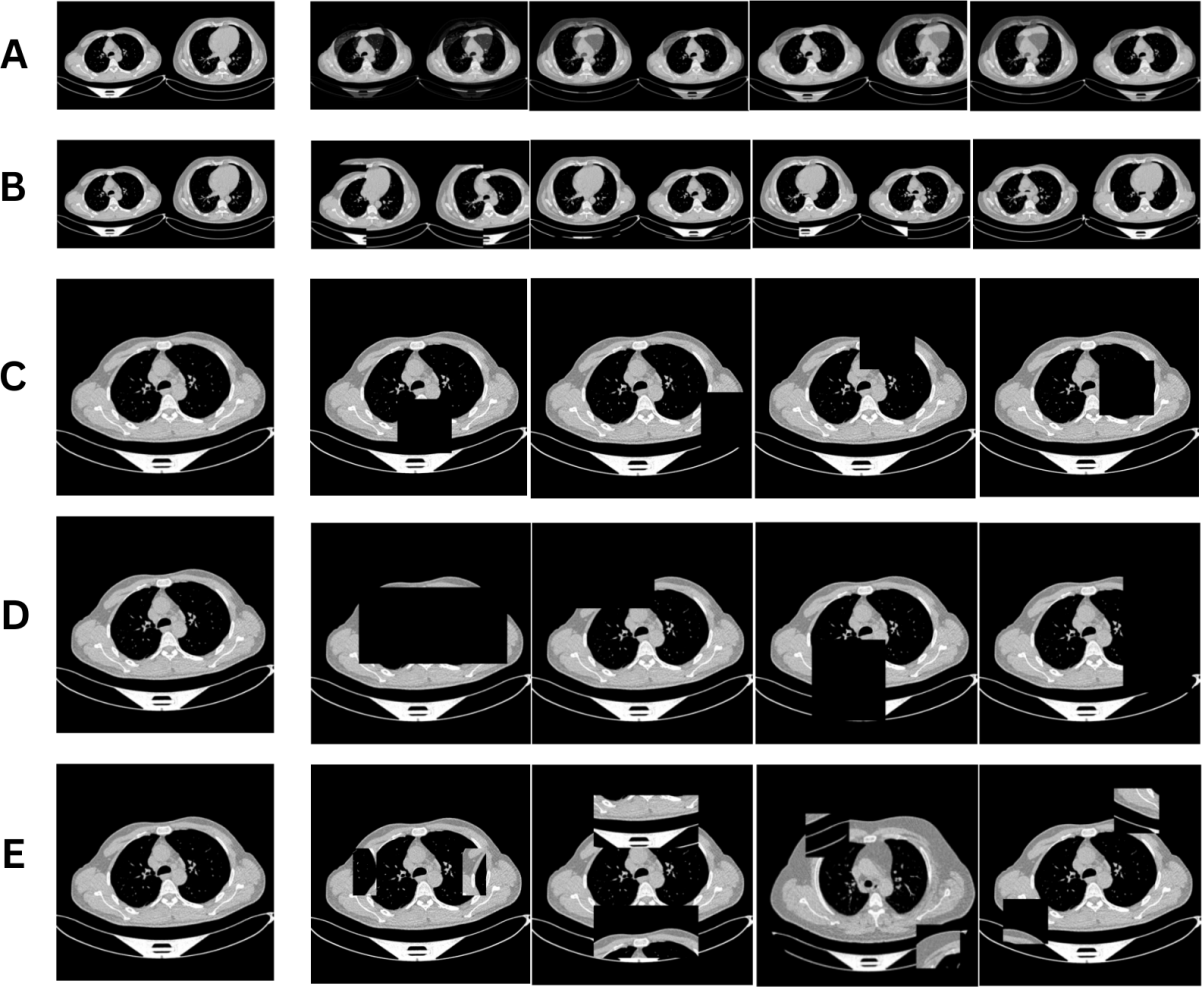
Computed tomography images for various data augmentation techniques. (A) MixUp; (B) CutMix; (C) Cutout; (D) Random Erasing; (E) Random Pixel Swap. The original image is in column 1, while the augmented images are in columns 2, 3, 4, and 5.
